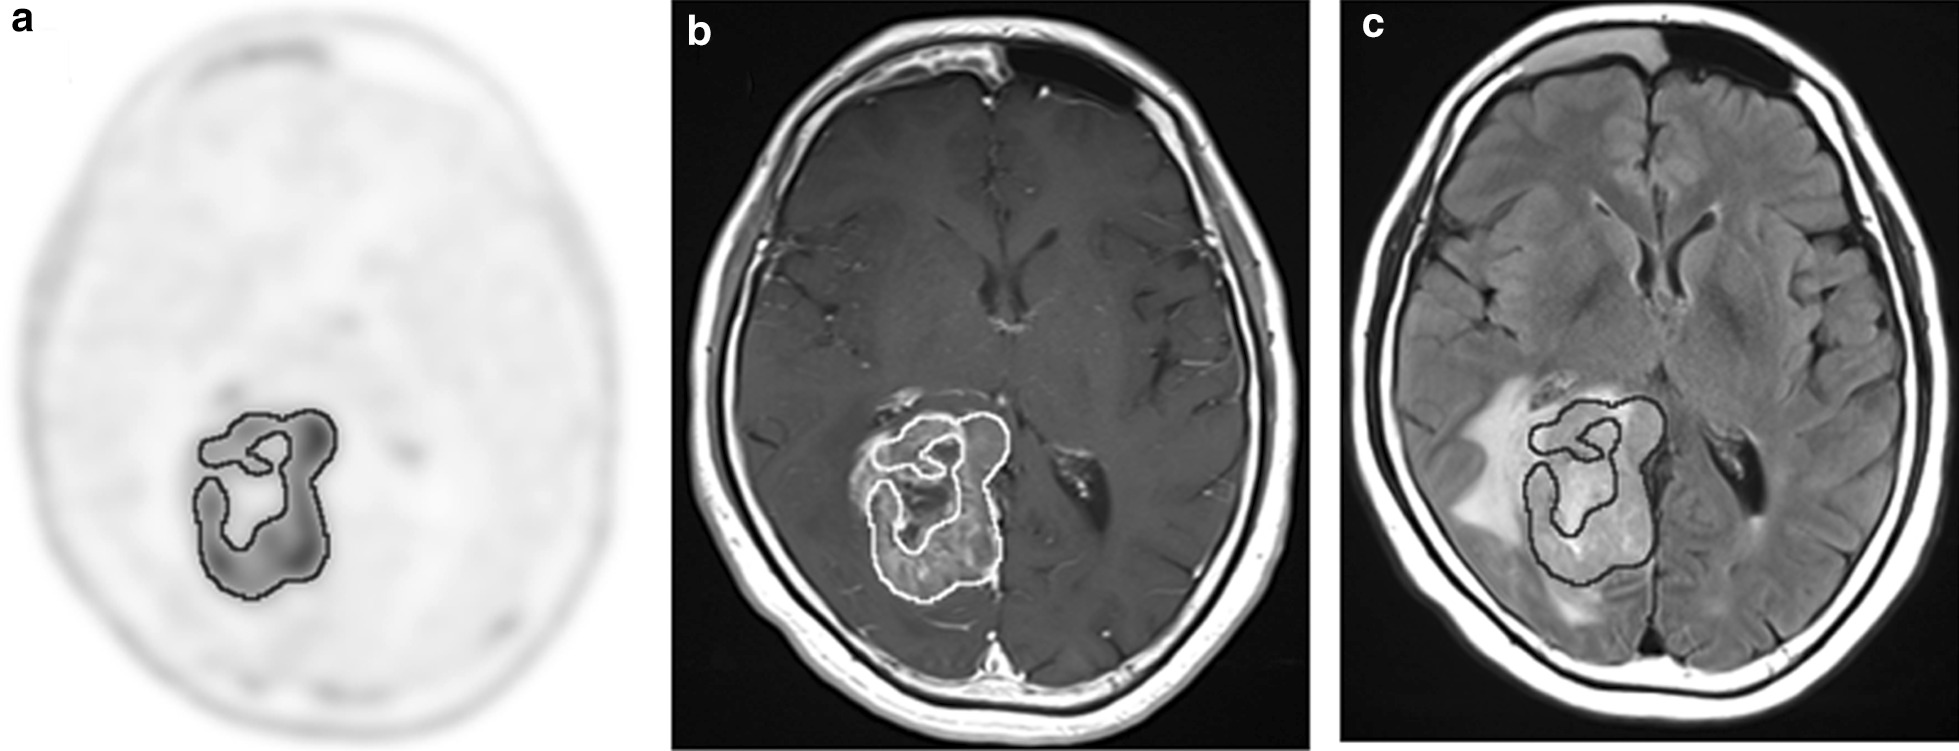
Radiologic images of a 60-year-old female with newly diagnosed glioblastoma. Transverse 4DST PET image a shows inhomogeneous uptake in the right occipital lobe (SUVmax = 5.36, T/N ratio = 13.74, PTV = 44.58 cm^3^). Transverse T1-weighted MRI with Gd enhancement b shows inhomogeneously enhanced lesion in tumor (GdV = 48.28 cm^3^). Region of interest shows proliferation area drawn on 4DST PET image from a. The proliferation area on 4DST PET image and Gd enhancement area on MRI differed very slightly. The hyperintense area identified on transverse FLAIR MRI c is larger than the proliferation area on 4DST PET image (FLAIRV = 137.52 cm^3^)
